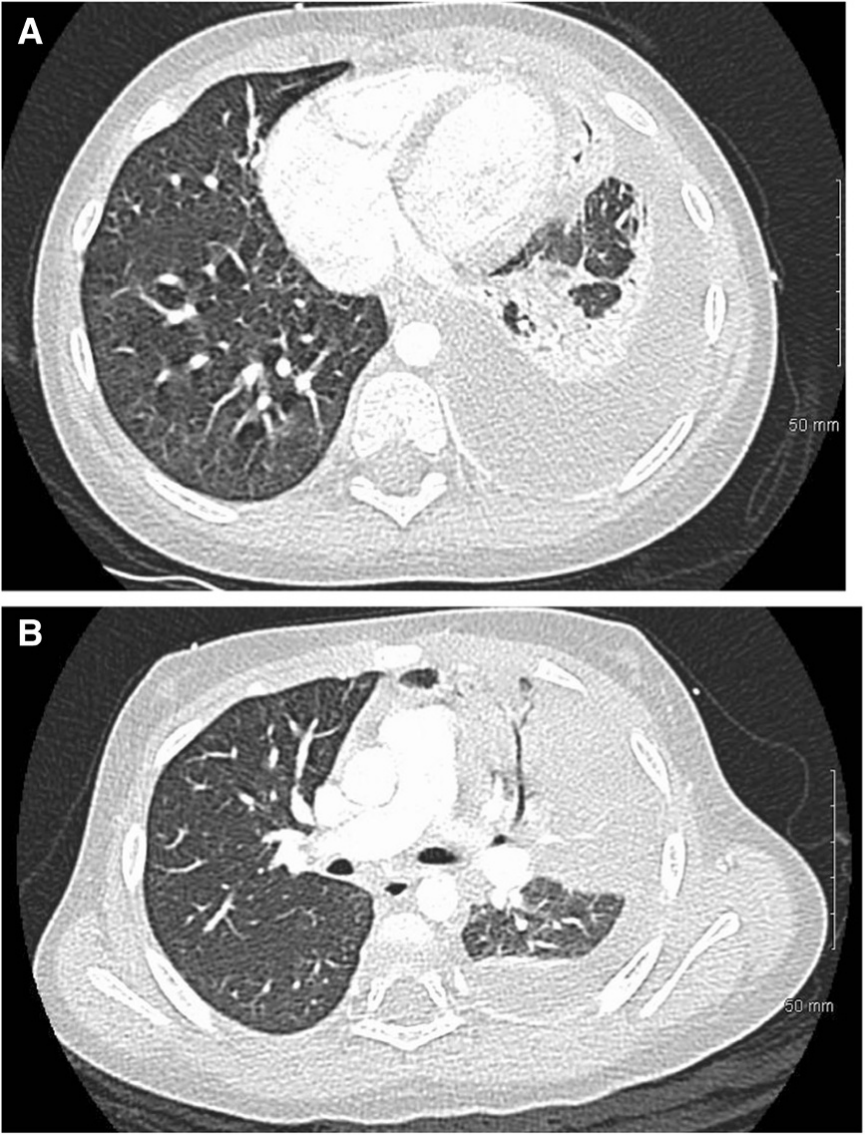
Images of CT scan of chest obtained from 2 year old child with empyema. A) Large pleural effusion with resulting left lower lobe compressive atelectasis. B) Left upper lobe consolidation with air bronchogram.
